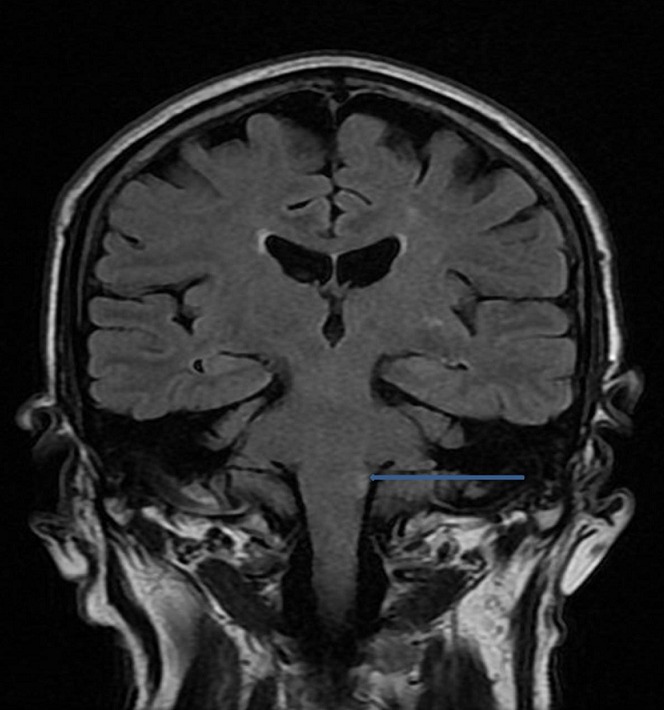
Coronal T2 FLAIR image showing hyperintense lesion in the right lateral medullary region
